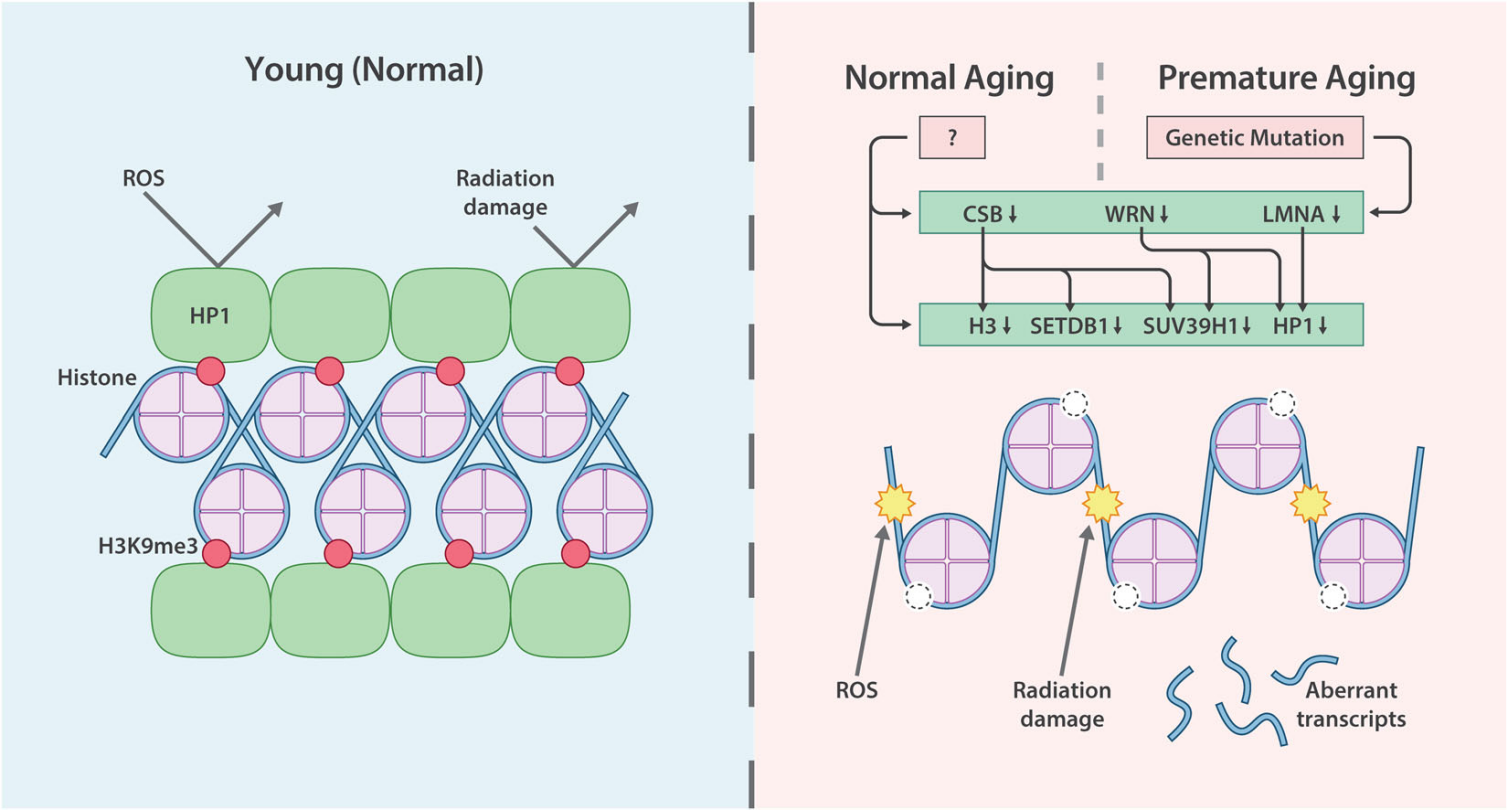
Overview of heterochromatin loss during aging. In young and healthy individuals, cells exhibit intact heterochromatin, a high level of H3K9me3, and a high level of HP1 bound to H3K9me3, which are factors that stabilize the heterochromatin complex. However, both chronologically and prematurely aged cells show decreased expression of core histones and reduced levels of H3K9me3 and HP1. CSB, WRN, and LMNA deficiency leads to H3, SETDB1, SUV39H1, and HP1 dysregulation, resulting in heterochromatin loss, DNA damage accumulation, and the expression of aberrant transcripts. The mechanisms under the decreased expression of CSB, WRN, and LMN with normal aging remain unclear.
